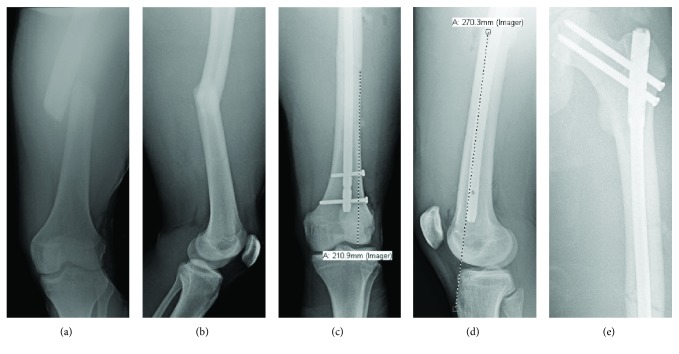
(a) AP radiograph of the left femur and (b) lateral radiograph of the left femur obtained upon patient presentation to the Emergency Department. (c) Postoperative AP radiograph of the left femur demonstrating fracture line 21.1 cm proximal to the center of the knee. (d) Postoperative lateral radiograph of the left femur demonstrating fracture line 27.0 cm proximal to the tibial tuberosity. (e) Postoperative radiograph of the left proximal femur.
